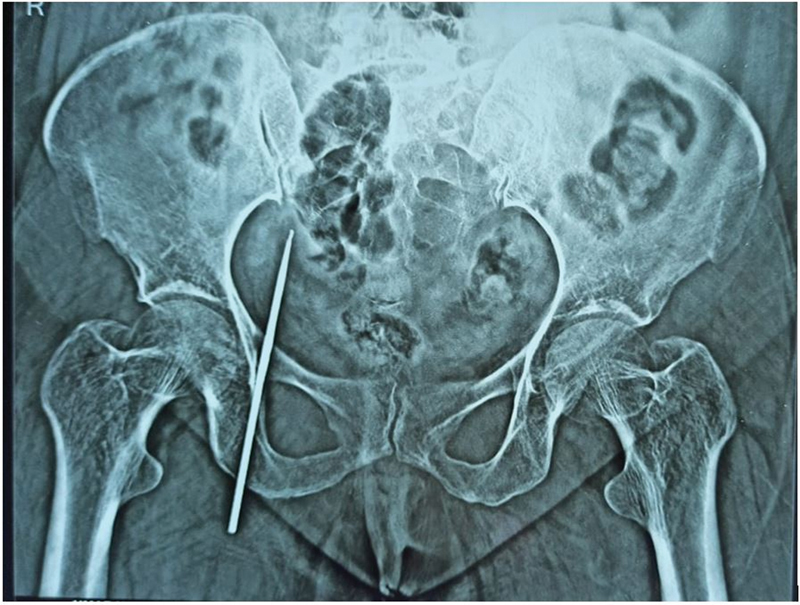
X-ray pelvis showing a linear radio-opaque shadow in the right hemipelvis.
